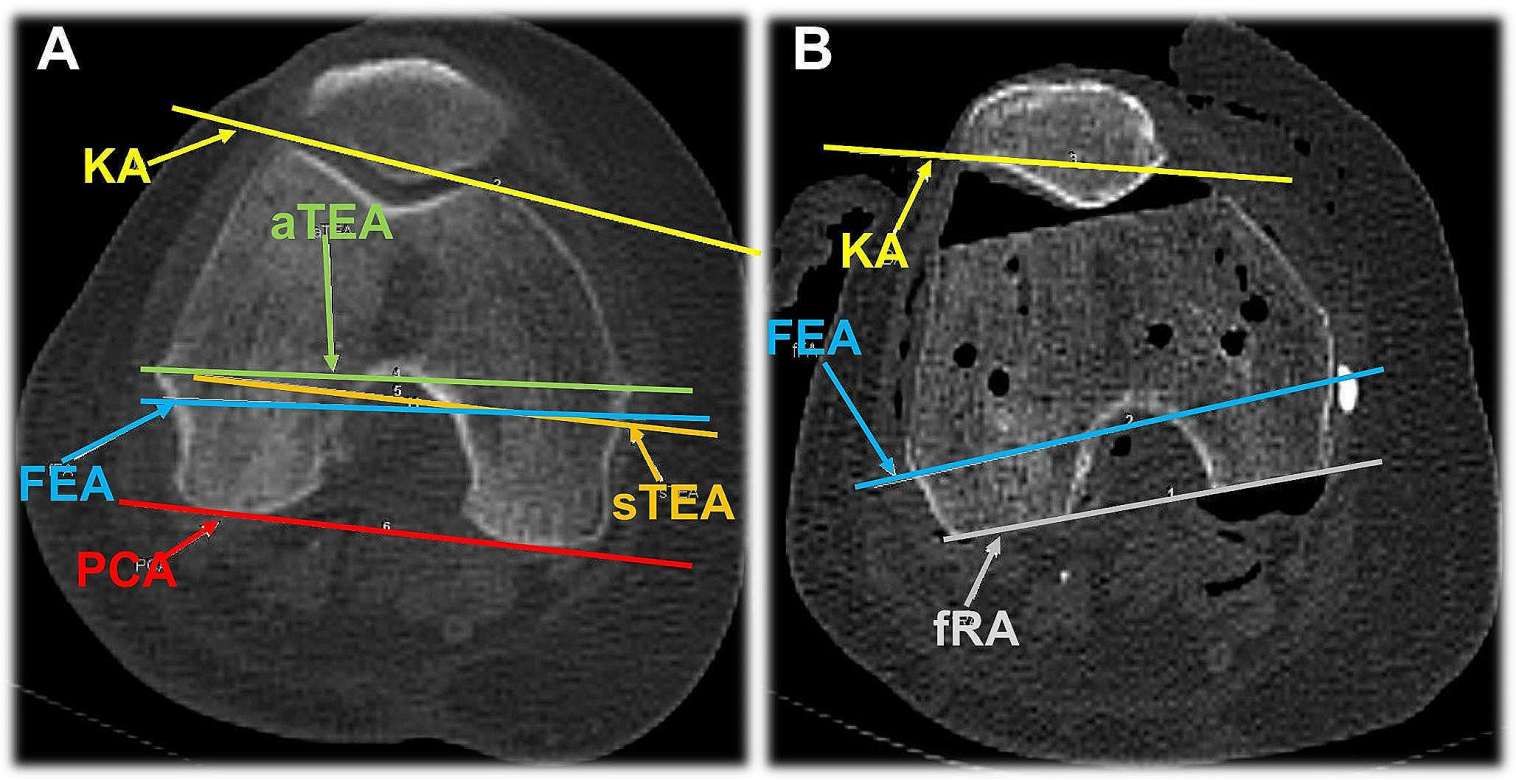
Axial CT imaging of the knee. (A) Preoperative determination of anatomic axes. KA = K-wire axis, aTEA = anatomic transepicondylar axis, sTEA = surgical transepicondylar axis, FEA = flexion-extension axis, and PCA = posterior condylar axis. (B) Postoperative axis determination. KA = K-wire axis, FEA = flexion-extension axis and fRA = functional rotation axis
